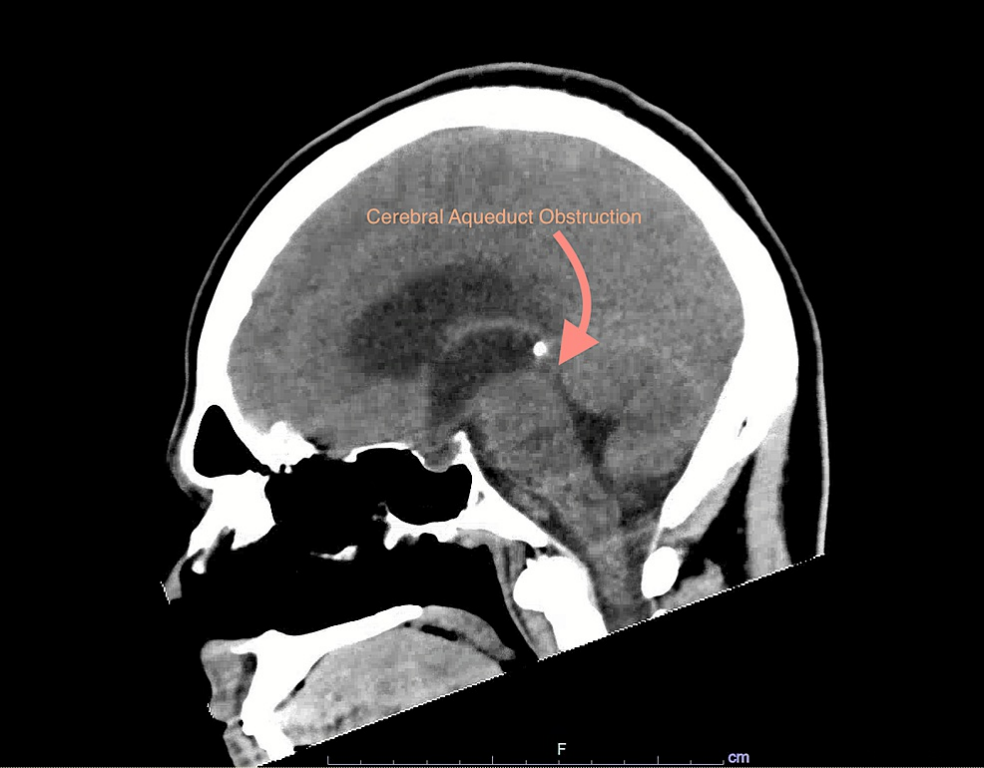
CT head/brain/cervical spine shows hydrocephalus with obstruction at cerebral aqueduct
